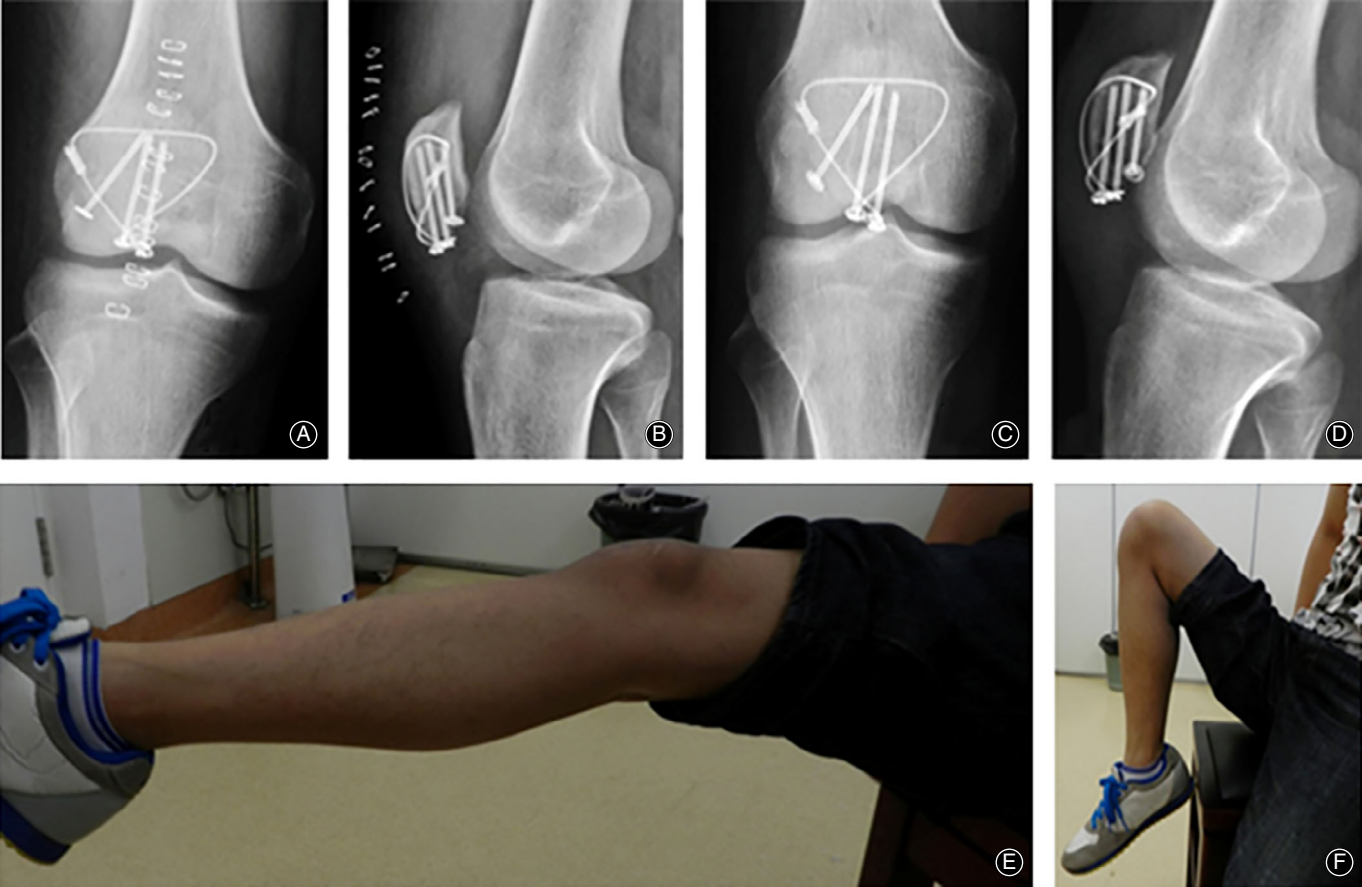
(A) and (B) Anteroposterior and lateral radiographs of the knee postoperatively. (C–F) A total of 2 months postoperatively, the fracture was well healed; the right knee joint functioned normally, and normal walking function was restored (Bostman score: 30 points).
